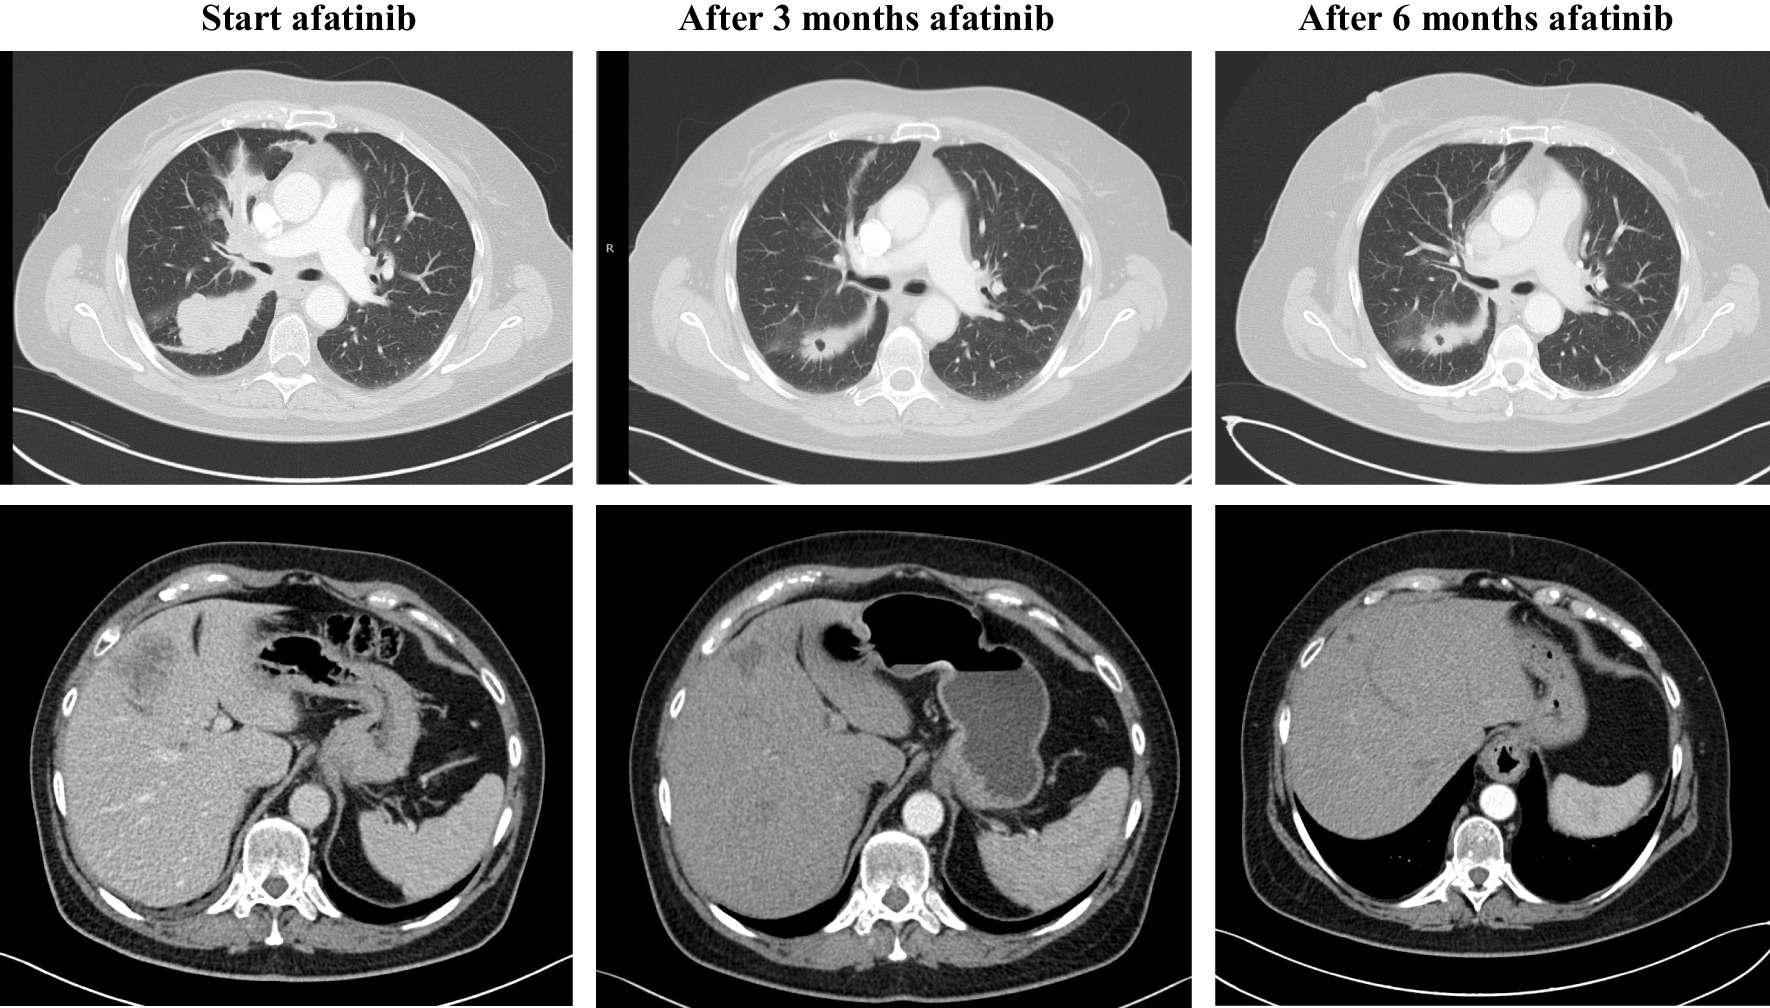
Radiographic response after afatinib. Standardized uptake value (SUV) lung lesion 10.8 g/ml
